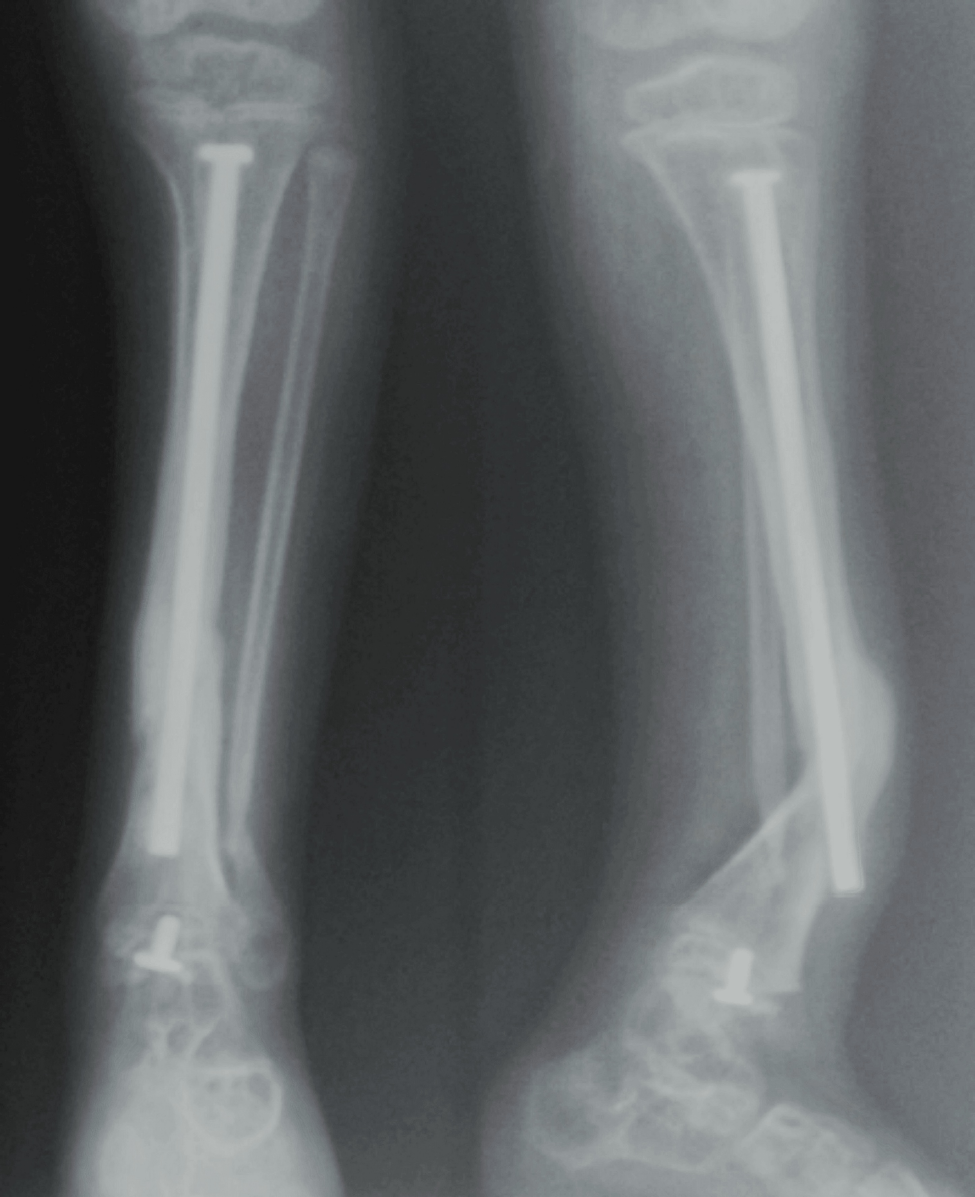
Radiograph showing T-piece migration in a telescopic nail This complication can affect the telescoping mechanism and lead to implant dysfunction.
